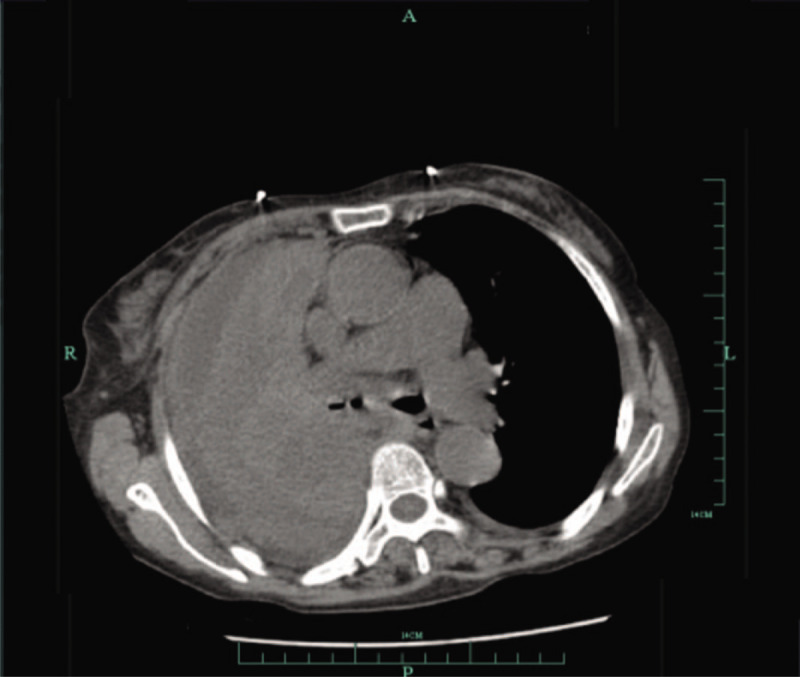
Chest CT scan showing right hilar soft-tissue mass associated with signs of congestive pneumonia and proliferative foci that had partial calcification in the right upper lobe.
